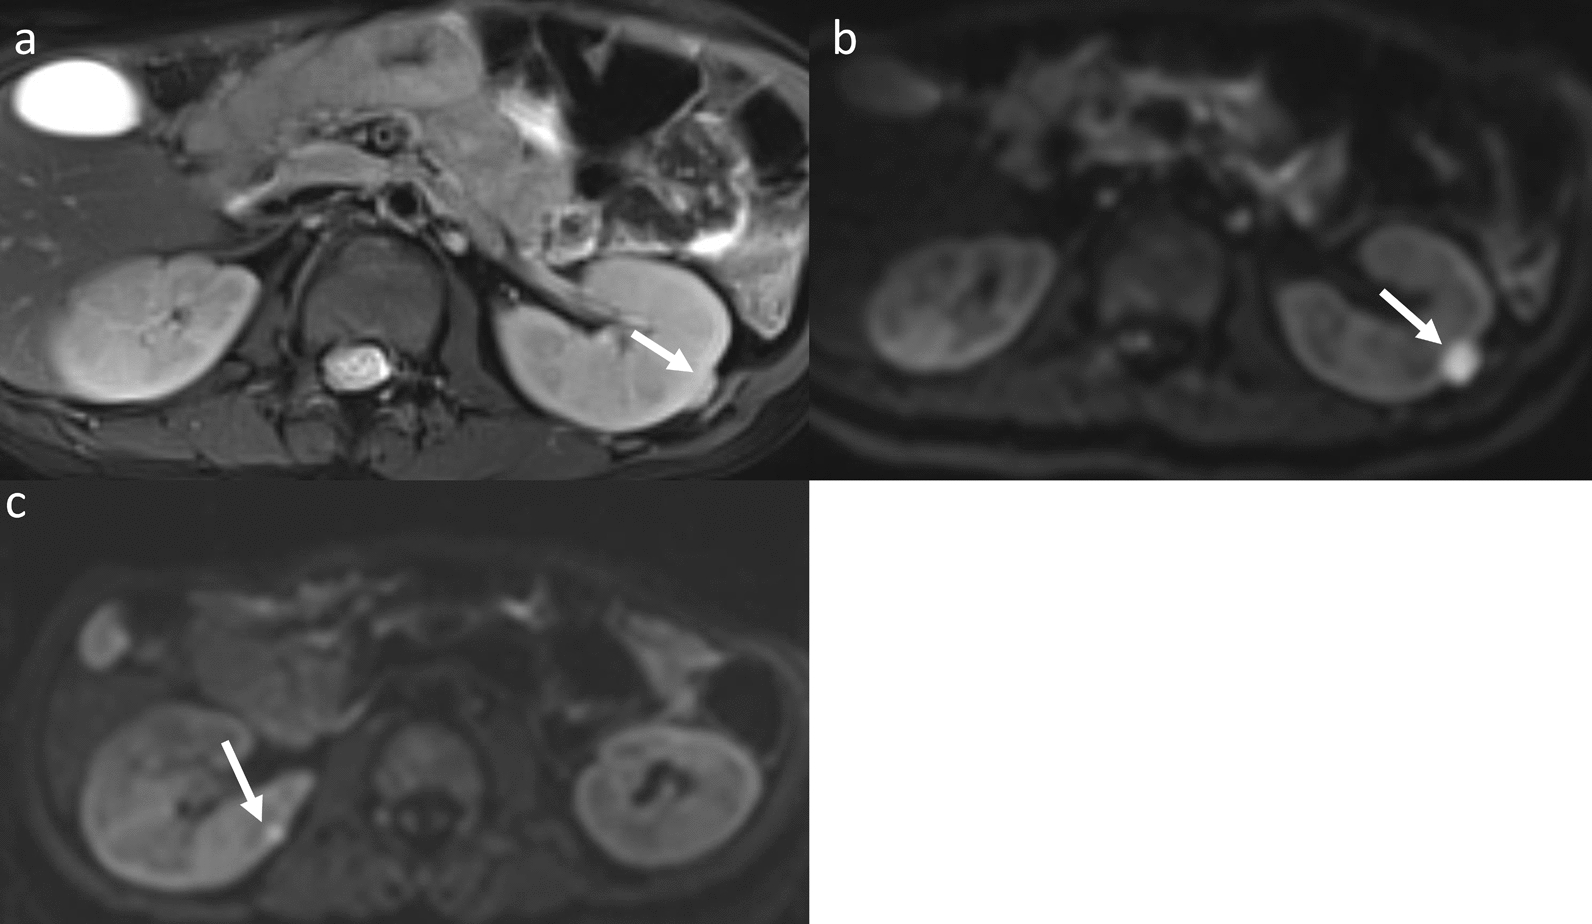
MRI of nephromegaly and complex cysts in a 2-year 3-month-old boy with BWS^IC1^ and a vascular malformation in the back. Arrows indicated an isointense signal on the fat-suppressed T2WI (a) and a hyperintense signal on the DWI (b) in the left kidney. A smaller hyperintense signal was observed in the right kidney on the DWI (c), which was not present on the T2WI
